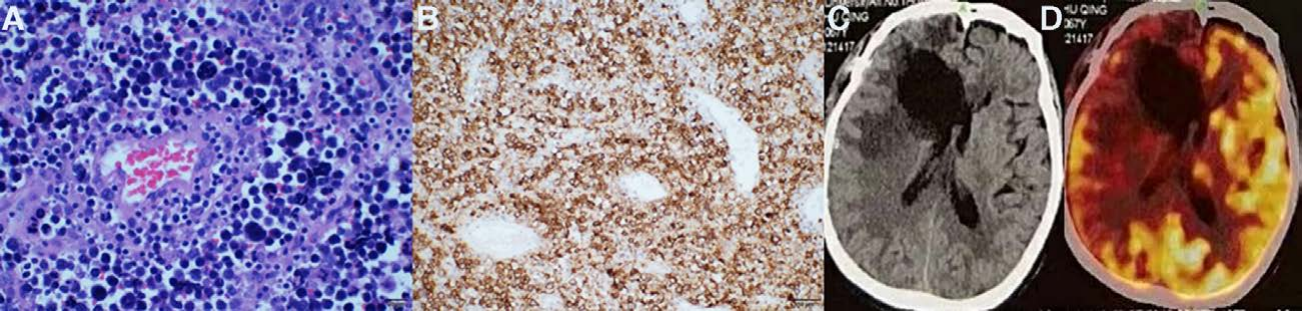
(A) The tumor cells were arranged in a cuff-like manner centered on blood vessels (HE; scale bar, 400×). (B) Tumor cells were CD79a + (scale bar, 200×). (C) Postoperative CT showed tumor disappearance. (D) No abnormal hypermetabolic uptake was seen on PET/CT at 19 mo postoperatively. PET/CT = positron emission tomography/computed tomography.
